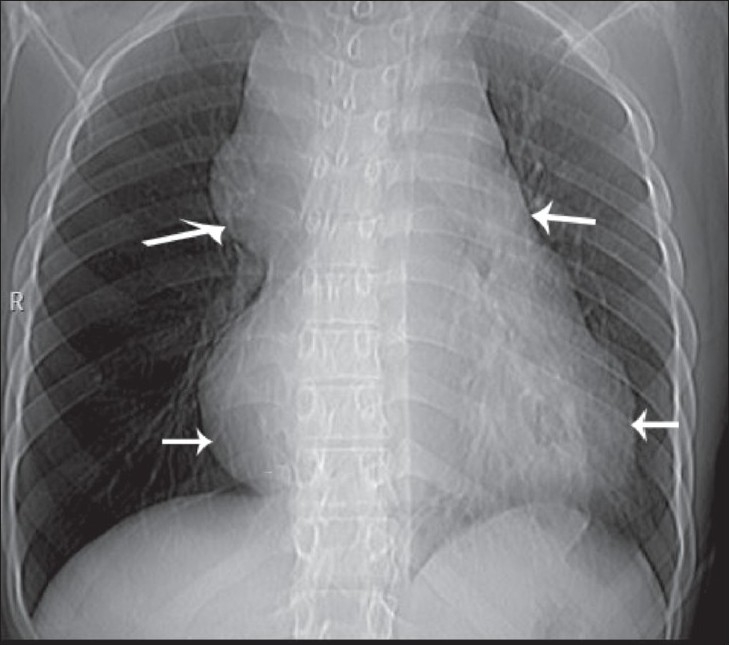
Frontal chest radiograph shows mediastinal widening (arrows)
